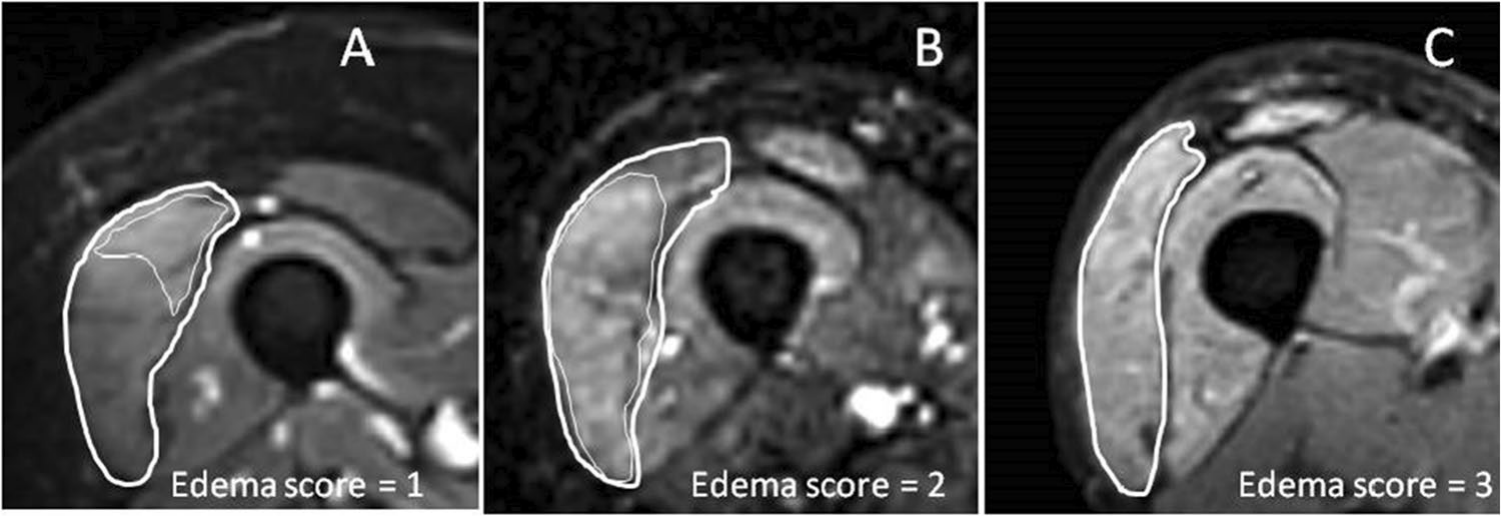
Axial EPI-T2-weighted sequences (TE = 90 ms) of the thigh depicting three different grades of edema in the right vastus lateralis muscle. A Edema was scored as 1 because of the slight involvement of 1/3 of muscle; the patient was negative for IIM and had a final diagnosis of FSHD genetically confirmed. B Edema was scored as 2 because it was moderated and involved 2/3 of the muscle area; the patient had a final diagnosis IIM. C Edema was scored as 3 since it involved the total muscle area; the patient had a final diagnosis of IIM
